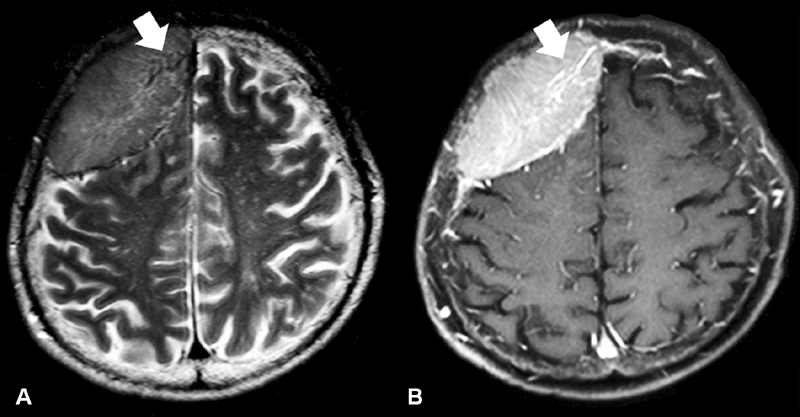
Magnetic resonance imaging on admission showing the vasculature of the tumor. There was a flow void sign on the T2-weighted image (
